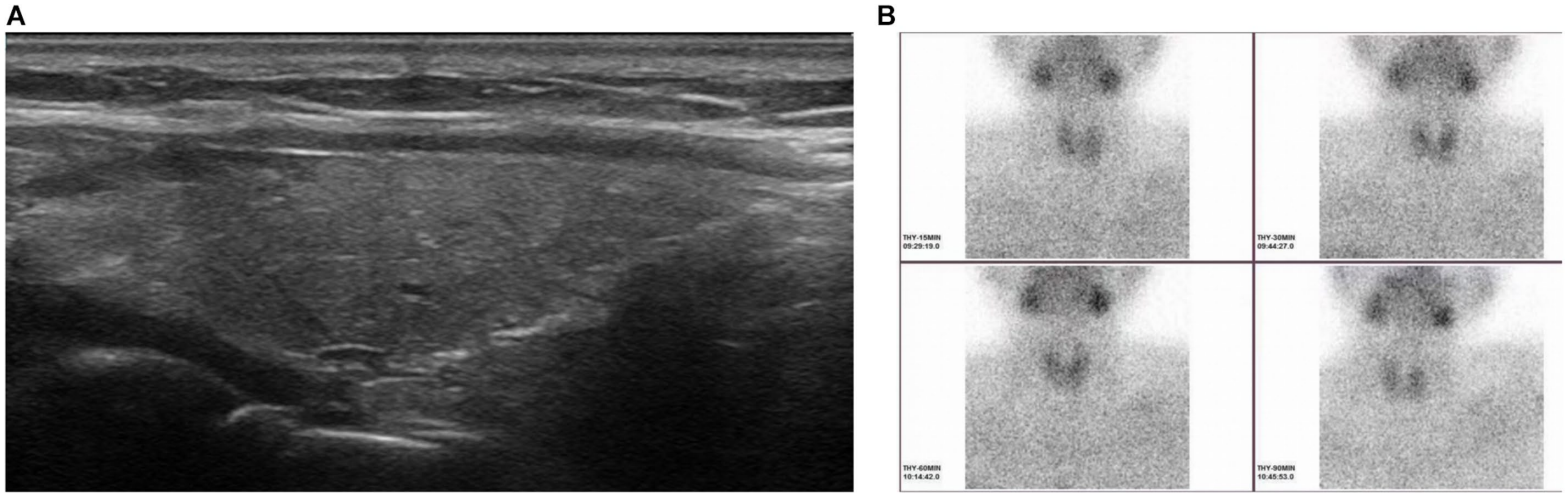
Ultrasound showing the primary hypoechoic area (parathyroid adenoma) disappeared 1 year after microwave ablation (A). No hyperplastic parathyroid was found in both the early and delayed phases (15, 30 and 60 min after the injection of methoxyisobutylisonitrile) when ^99m^Tc-methoxyisobutylisonitrile was performed 1 year after microwave ablation (B).
